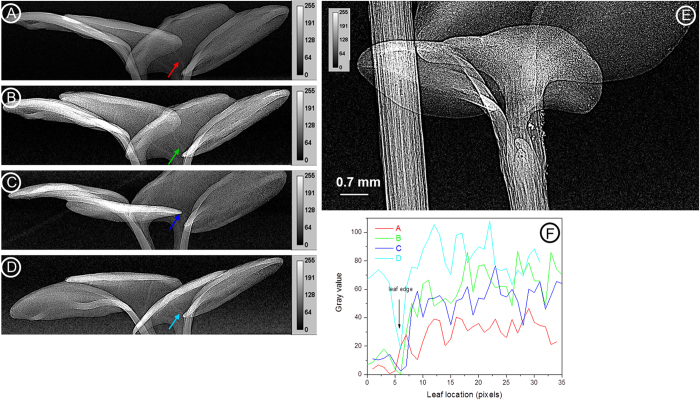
Selection of optimal X-ray energy for PCI of above ground plants. Images were recorded using an effective pixel size of 8.75 μm at 15, 18, 20 and 24 keV (A–D). The PC image of a canola plant meristem (E). Line profiles showing the phase signal histogram of marked regions in (A–D) (F). Note the movement of plant during imaging time from A to D.
